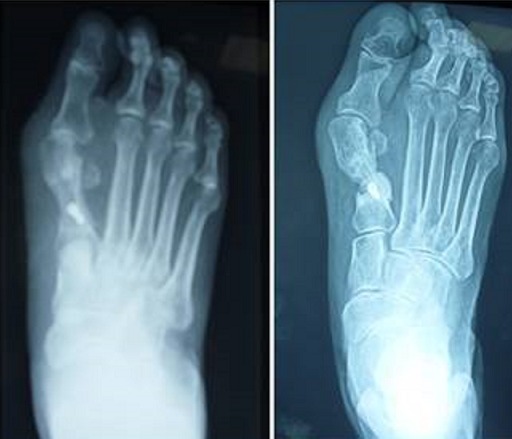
Le seul cas d'ostéonécrose: à gauche radiographie postopératoire après 2 mois et à droite radiographie après 8 mois de recul
